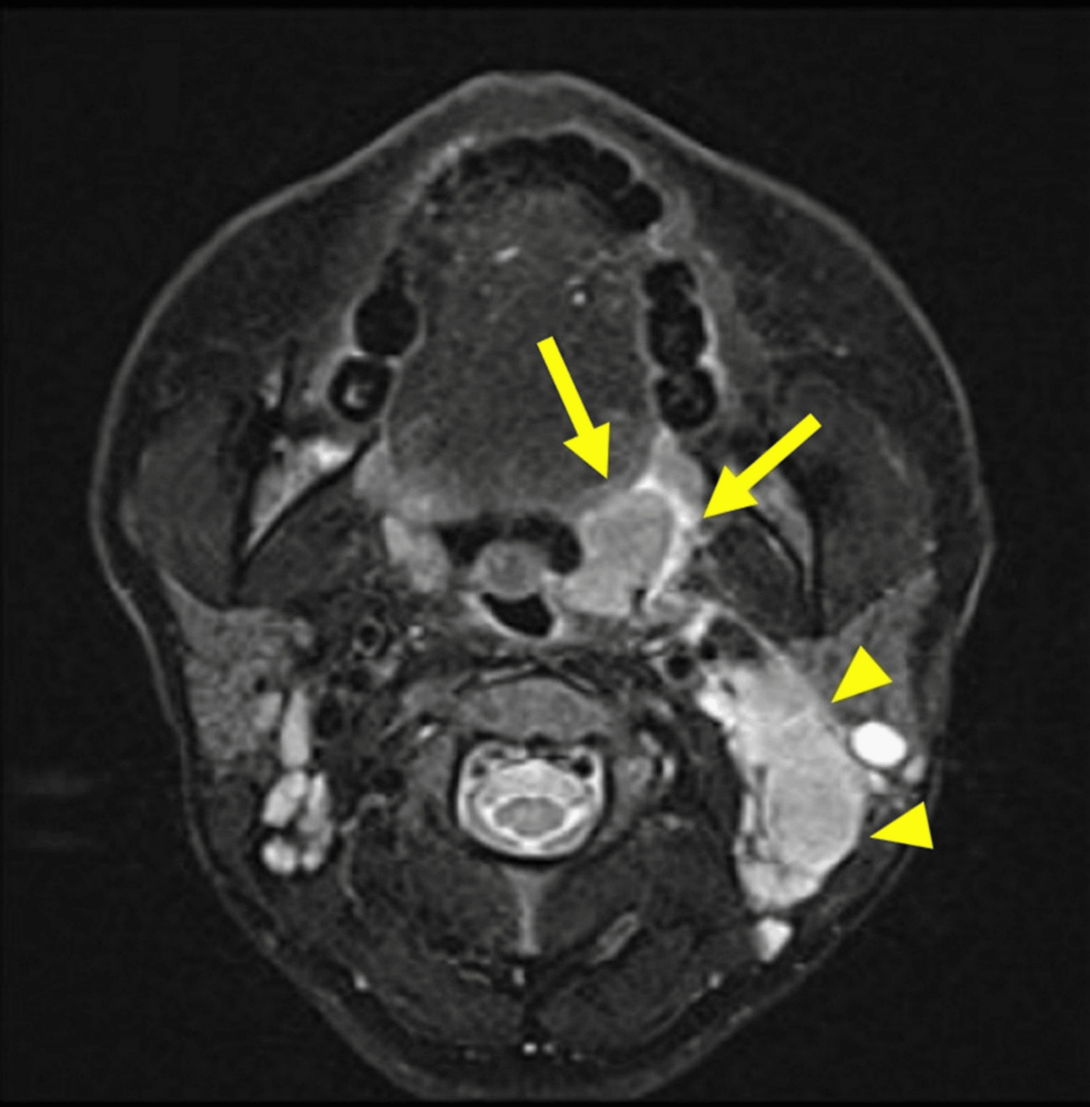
Axial fat-suppressed T2-weighted MRI image at presentation. A tumor measuring approximately 25 × 16 mm was identified in the left palatine tonsil (yellow arrow), along with multiple enlarged lymph nodes in the left cervical region, the largest measuring up to 5 cm (yellow arrowhead). The patient was diagnosed with left-sided oropharyngeal cancer originating from the palatine tonsil. The tumor was p16-positive and classified as clinical T2N1M0, Stage I, according to the TNM classification of Malignant Tumors, 8th edition [10].
